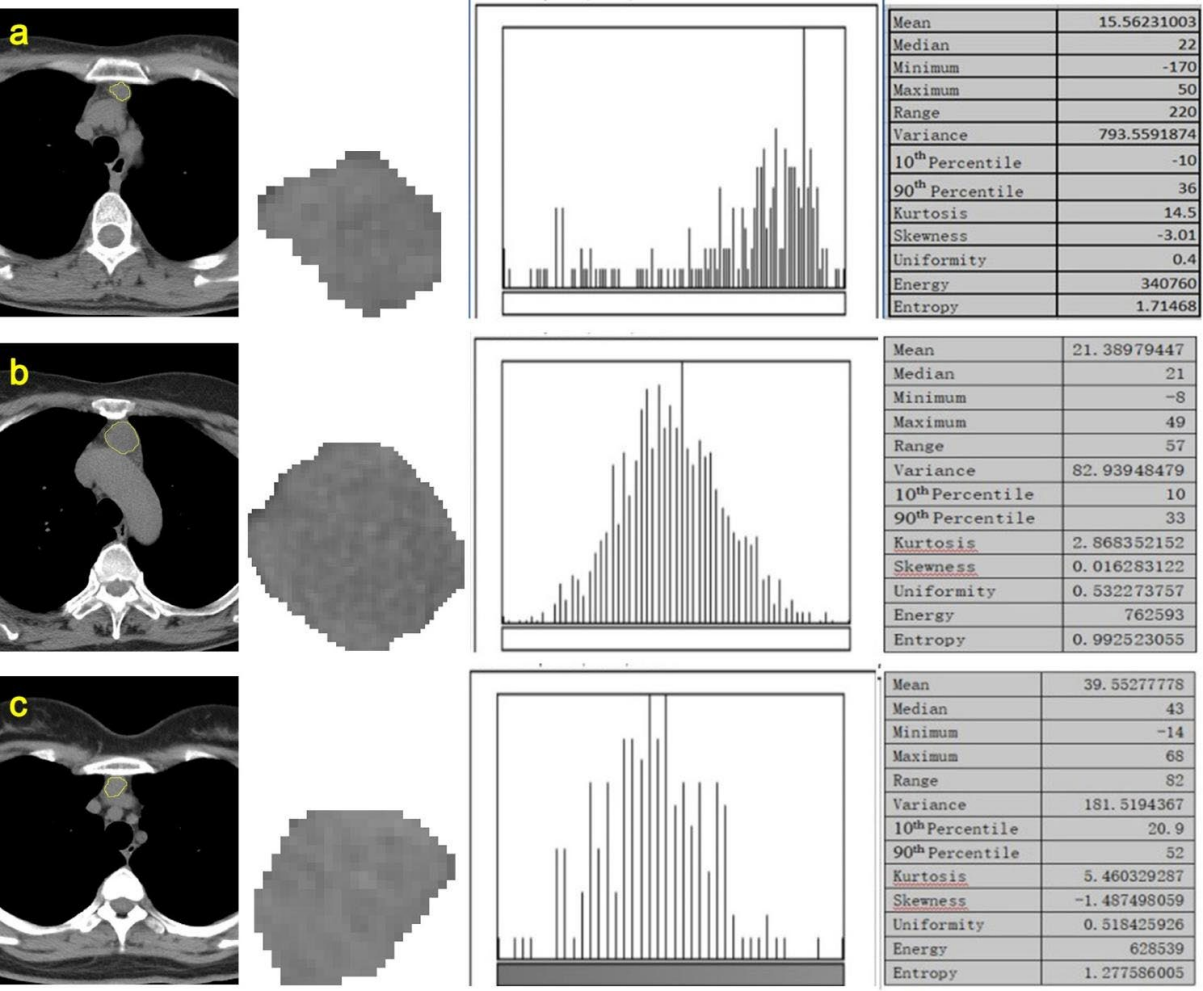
Axial unenhanced CT images, ROI setting, pixel intensity distribution histogram and histogram parameters of representative cases (a, thymic hyperplasia; b, thymic cyst; c, thymoma)
